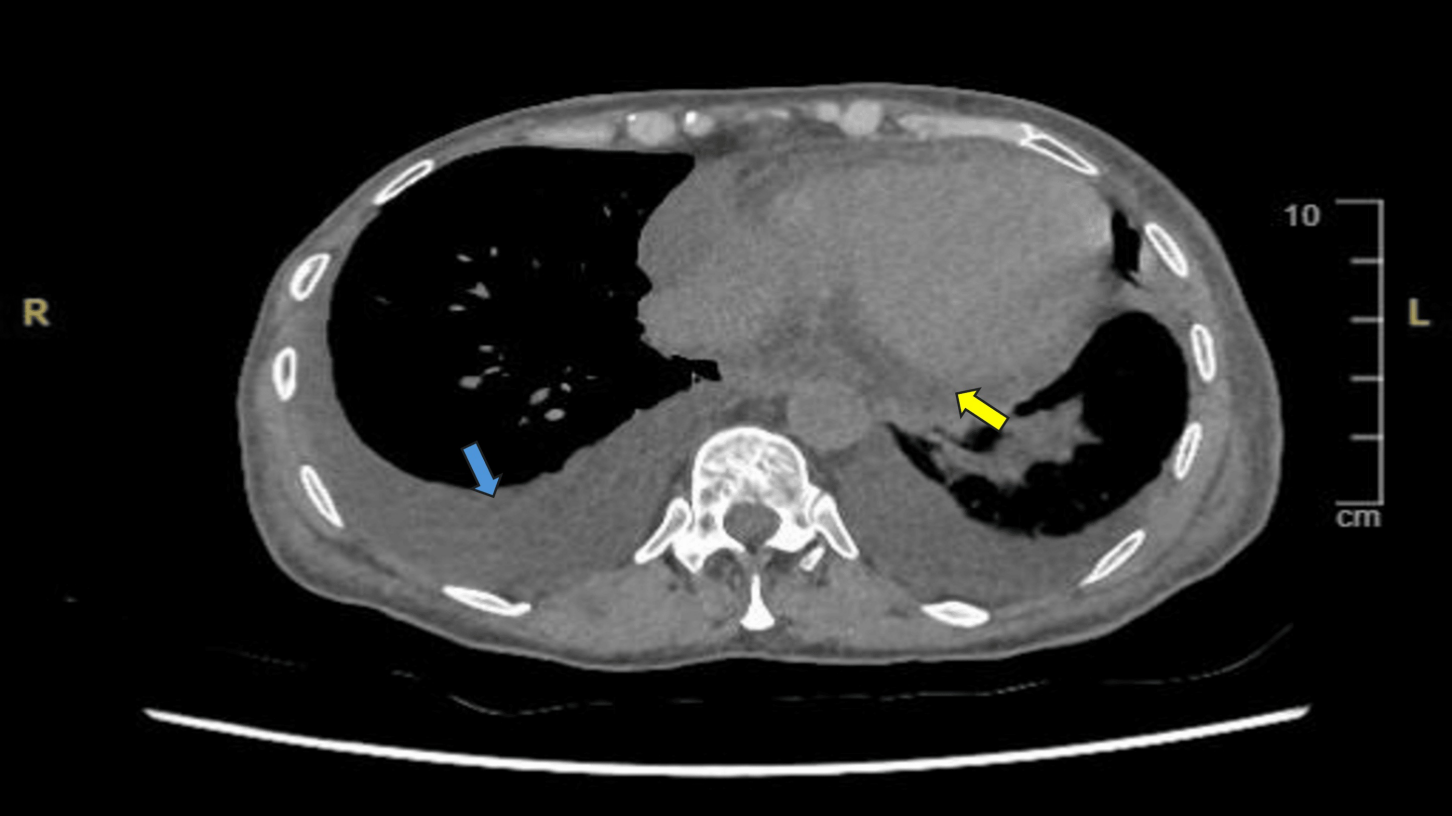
CT KUB of the patient (chest view) The blue arrow indicated pleural effusion. The yellow arrow indicated pericardial effusion. CT KUB: computed tomography of the kidneys, ureters, and bladder
